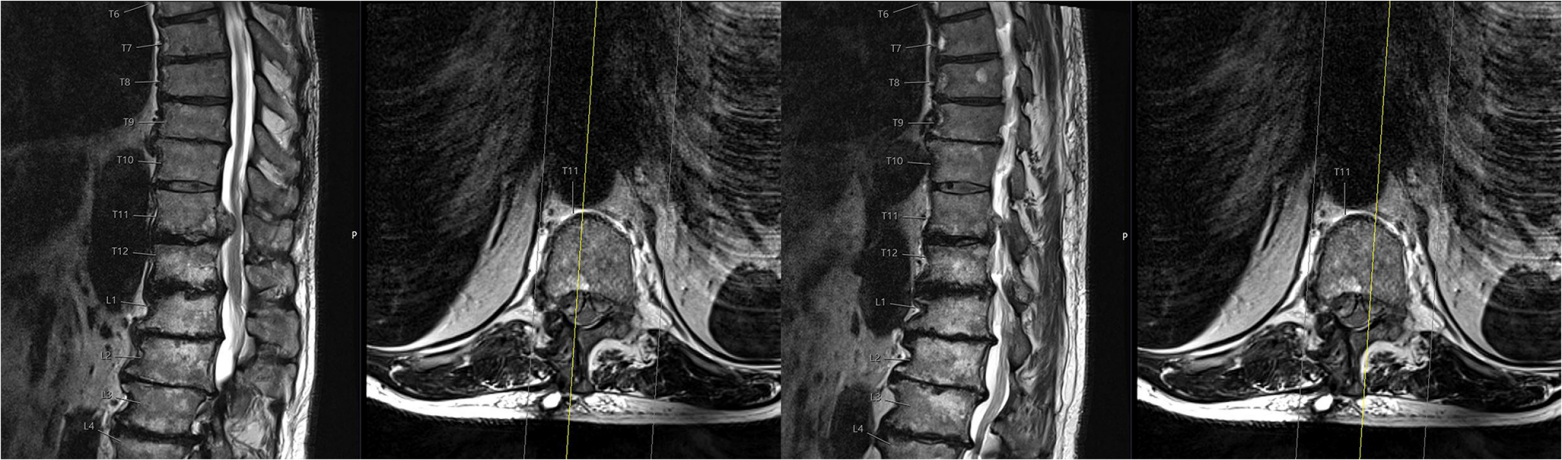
MRI of the thoracolumbar spine T2 weighted 8 weeks after initial presentation with clinical bilateral thoracic abdominal wall bulging and a bilateral relapse of disc herniation Th11/12
